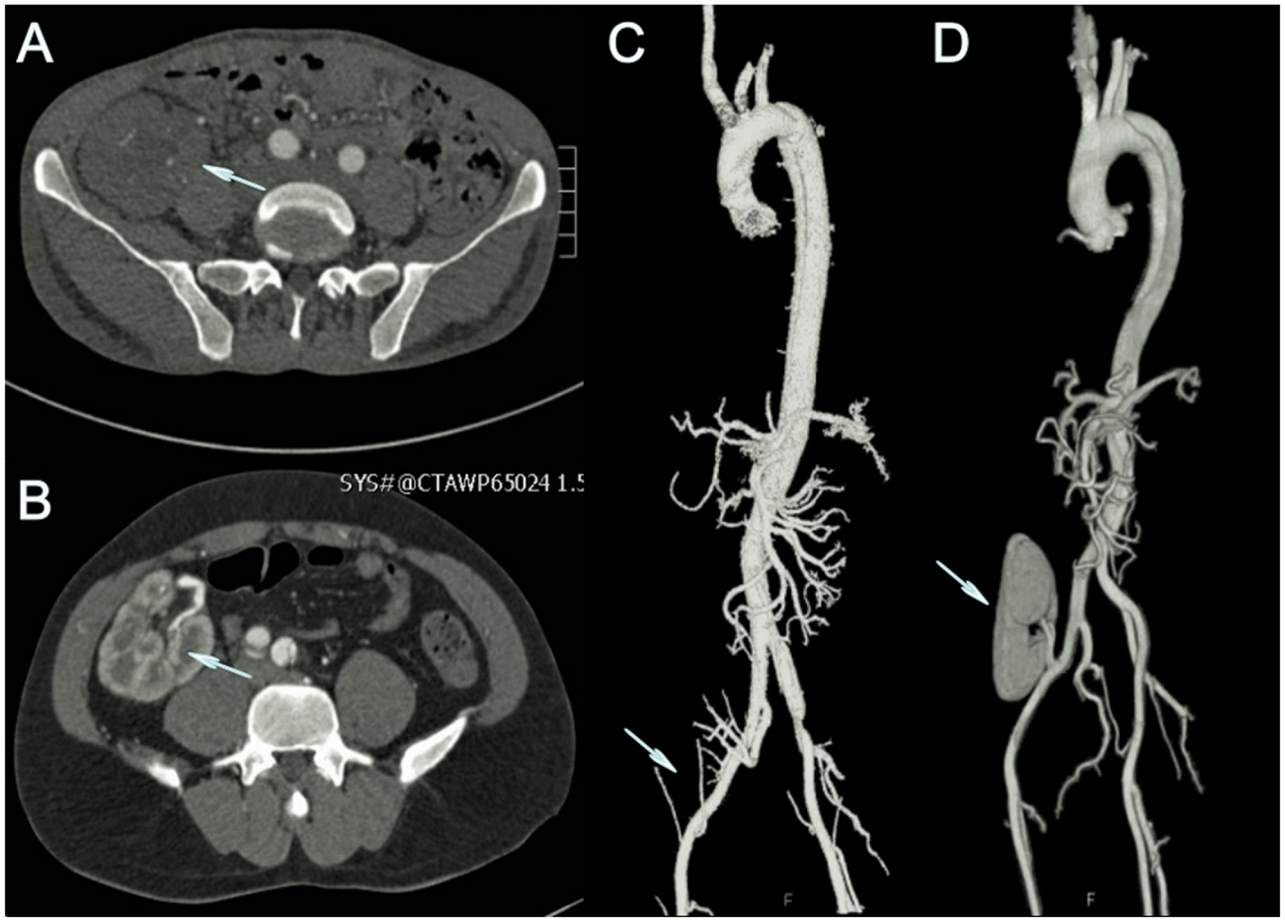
Comparison of preoperative CTA results between patient 2 and patient 1 (A+C): Preoperative CTA of patient 2 showed that no perfusion existed in the renal graft of this patient. (B+D): Preoperative CTA of patient 1 showed that the renal graft had normal blood perfusion.
